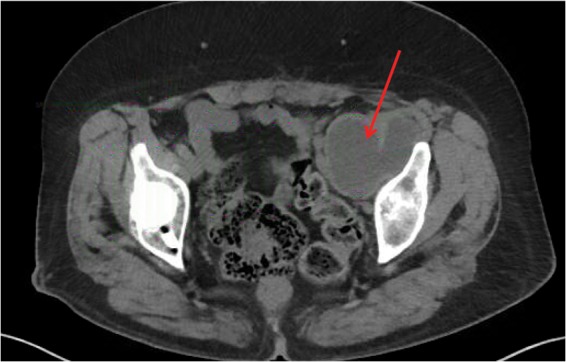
Axial cut from CT scan showing large fluid collection (arrow) measuring 5.5 × 6.0 × 10.4 cm that is originating from THA and displacing external iliac vasculature. CT, computed tomography.
